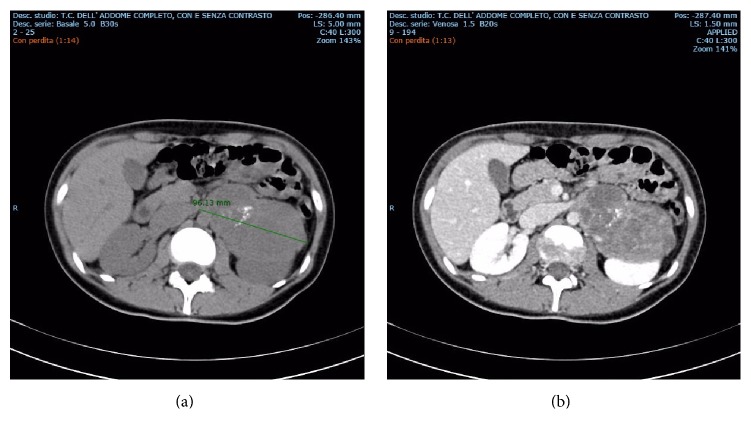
Abdominal CT scan with and without contrast enhancement: presence of a big and heterogeneous mass with calcification in left adrenal lodge (b). Dynamic analysis revealed a progressive and modest contrast enhancement in venous phase (a).
